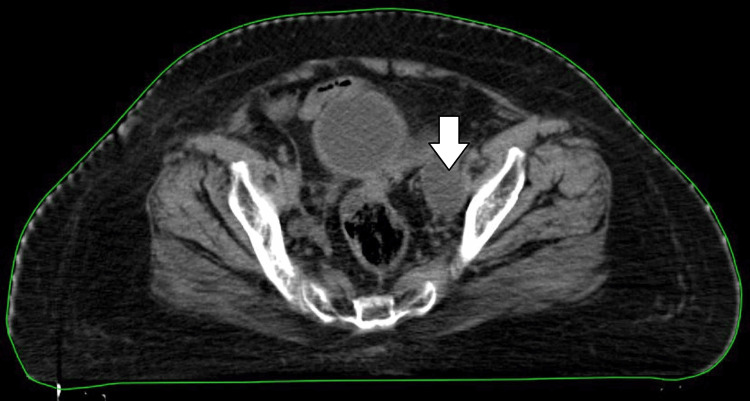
Post 10 fractions adaptive planning CT showing significant lymphocele volume reduction CT: computed tomography
